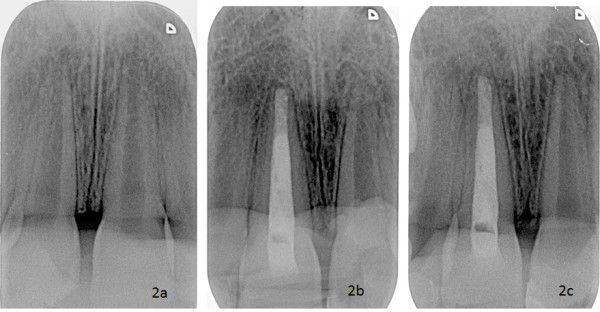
The reproducibility of the radiographs taken at each session allowing us to compare them. 2a: Radiograph taken during the inclusion session. 2b: Radiograph taken at the 6th month session. 2c: radiograph taken at the 12th month session.
